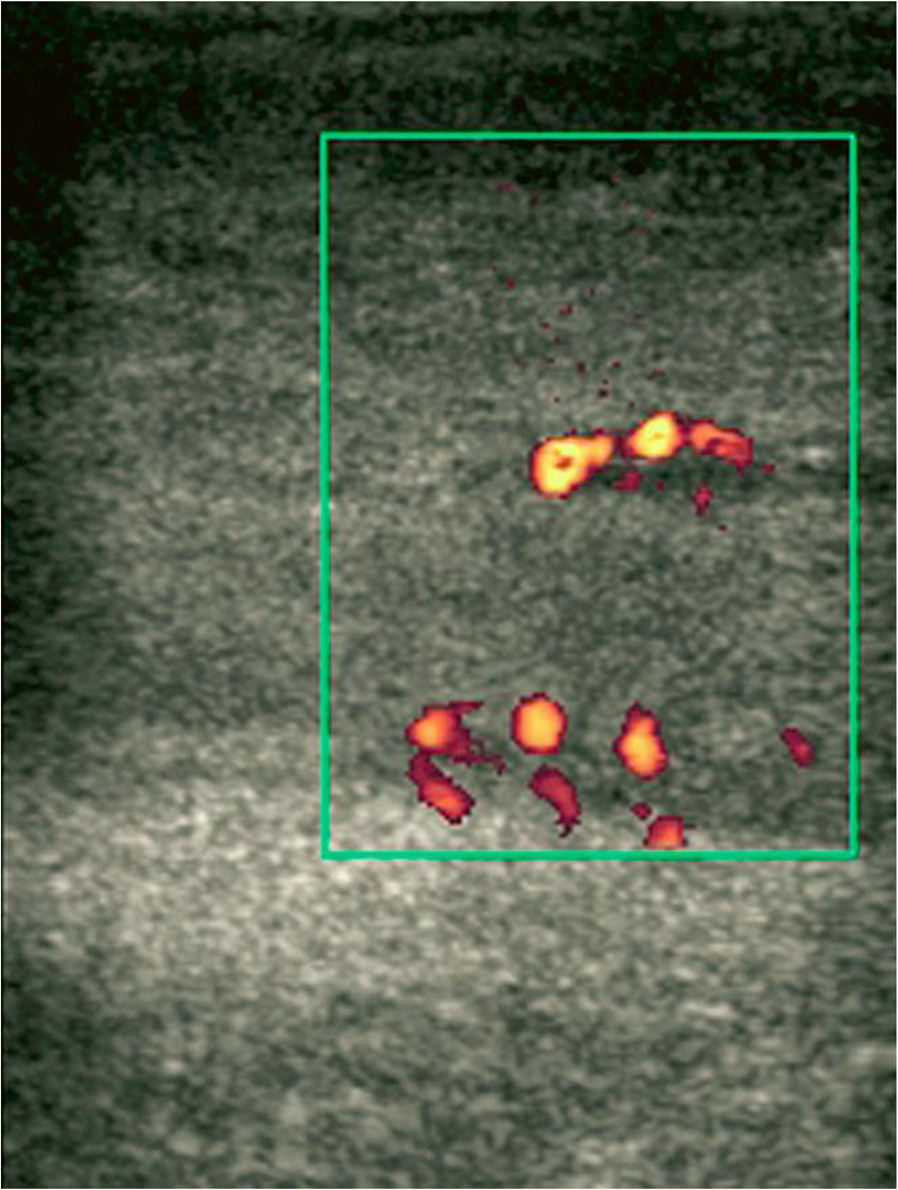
Power Doppler Ultrasound image showing blood flow within a lesion[2].
